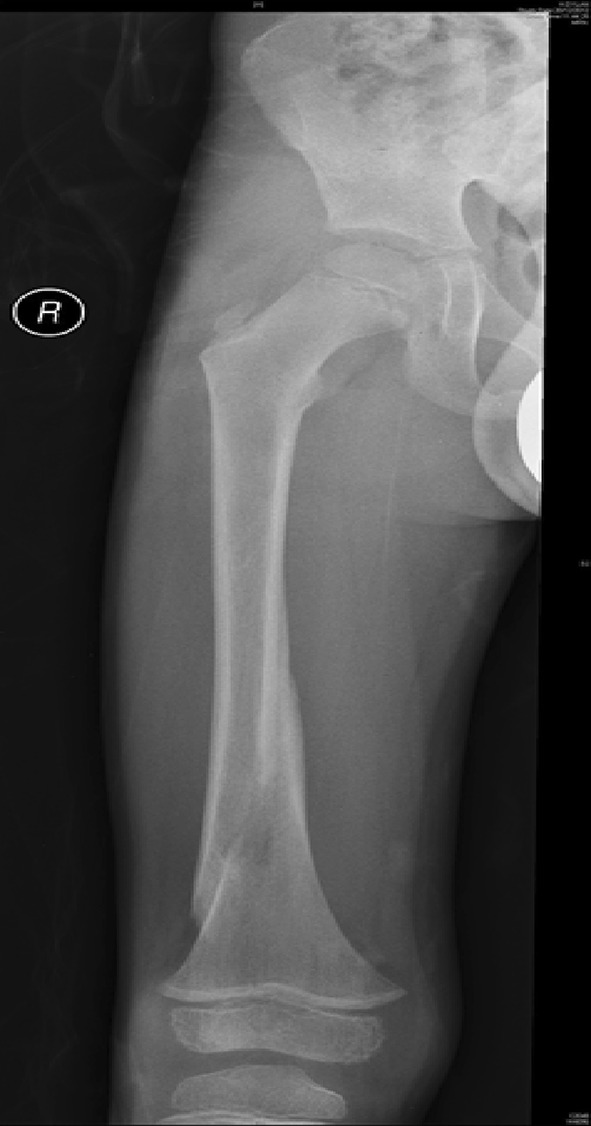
AP radiograph of the same child as shown in Fig. 2, taken at 8 weeks, showing solid union and acceptable alignment after an initial treatment of 3- to 4-week in-line traction
